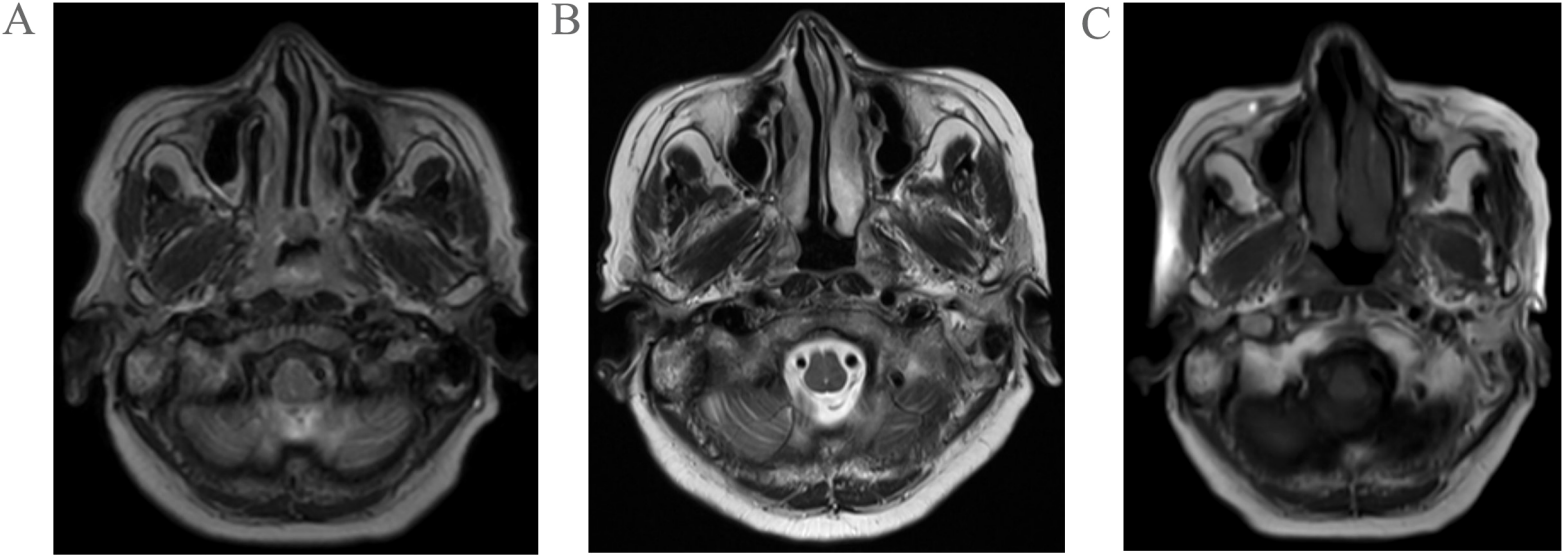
Pretreatment MRI findings of the patient (A); Post-2-cycle chemotherapy MRI findings of the patient (B); Post-1-cycle radiotherapy combined with nimotuzumab targeted therapy MRI findings of the patient (C).
